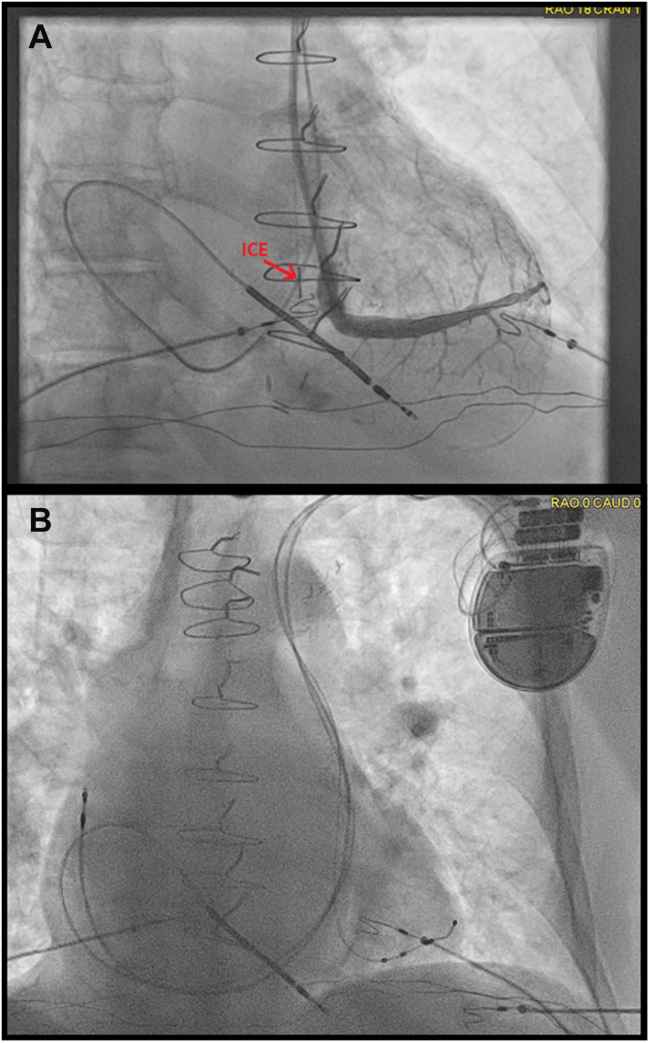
A–B: Intracardiac echocardiography catheter positioned within the coronary sinus body during selective venography of a lateral branch and final fluoroscopic imaging demonstrating optimal lead placement.
